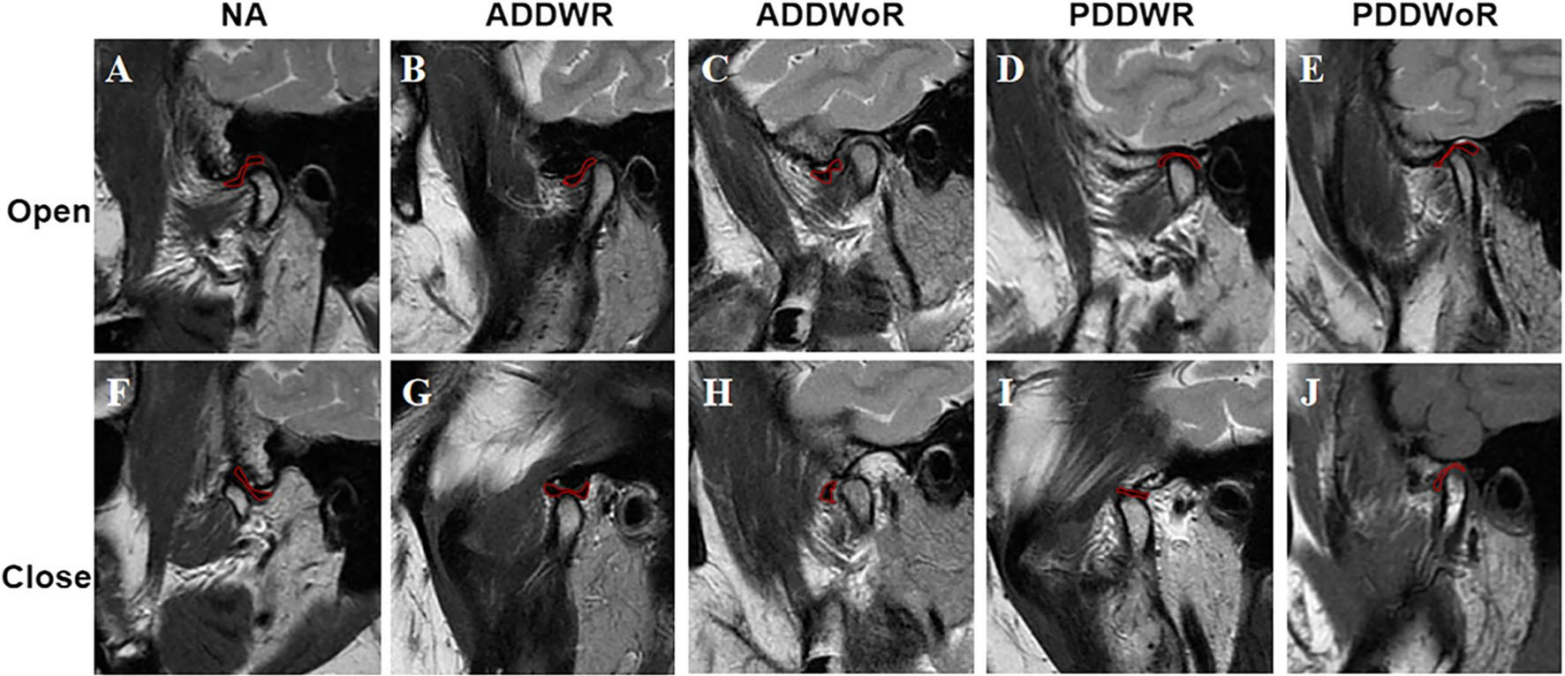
Disc position. A–E MRI images in the open-mouth position, F–J MRI images in the open-mouth position. Where: NA: normal; ADDWR: anterior disc displacement with reduction; ADDWoR: anterior disc displacement without reduction. PDDWR: posterior disc displacement with reduction; PDDWoR: posterior disc displacement without reduction
